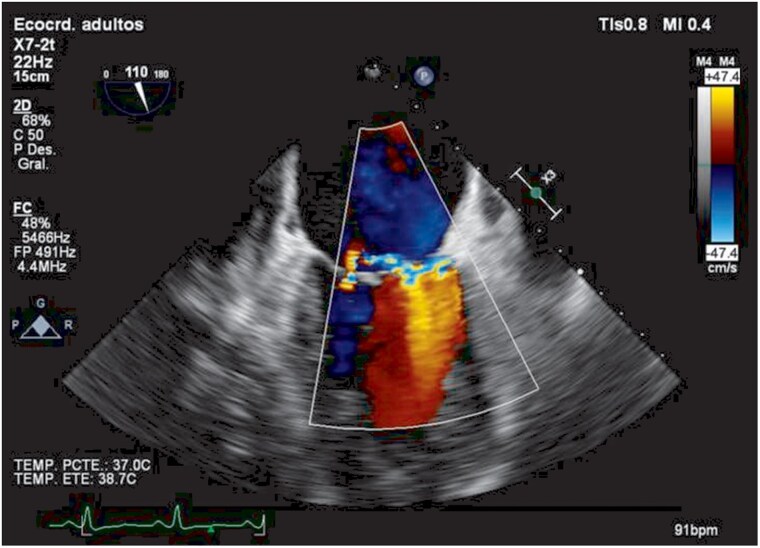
Transoesophageal echocardiography with colour Doppler showing anterior mitral leaflet perforation at segment A2 with a small regurgitant jet at the perforation site, together with a central jet of mitral regurgitation.
